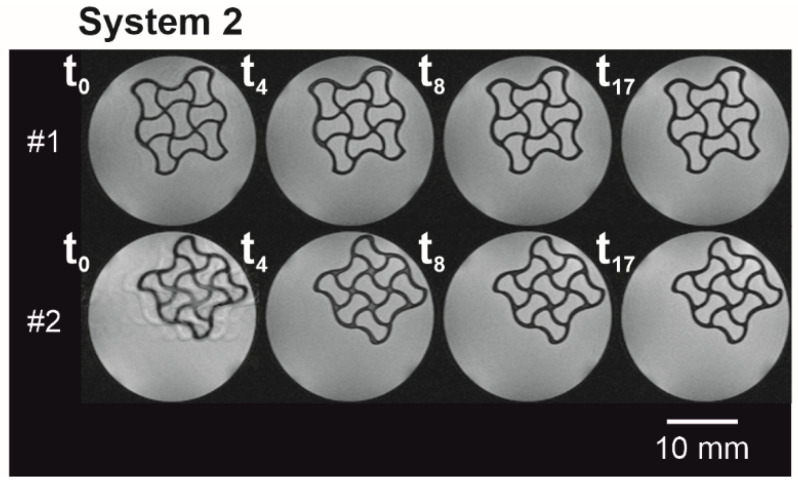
Auxetic structures in System 2 in MRI grayscale images with a field of view of 25 × 25 mm² at distinct time points. The MRI measurements’ settings were optimized towards an increased buffer-to-hydrogel contrast (*n* = 2).
